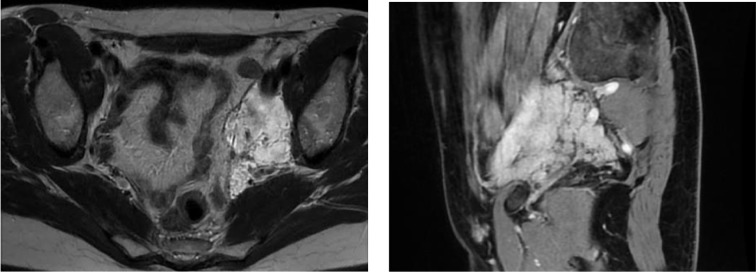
MRI: left iliac region. Suspicion of LAM
